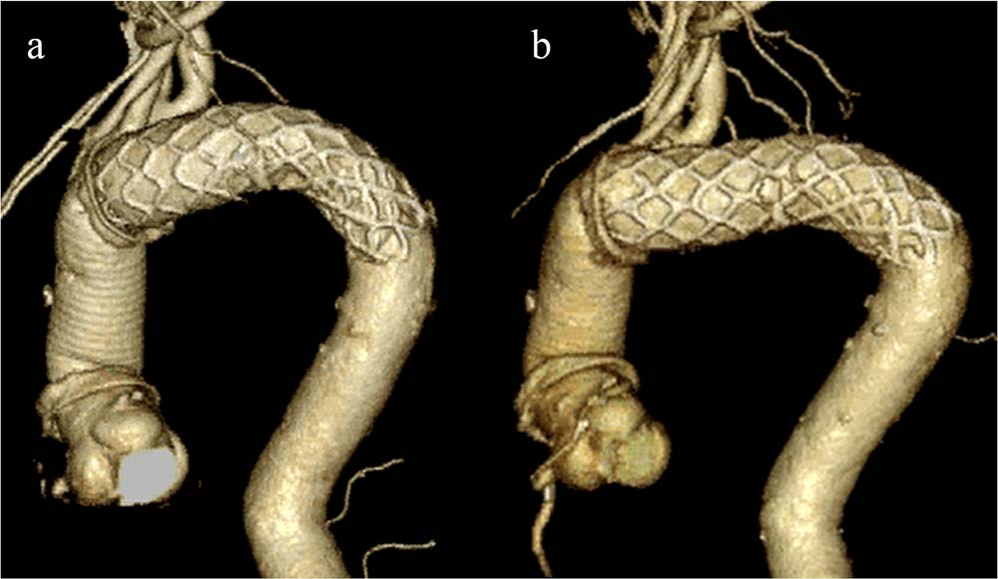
(a) Three-dimensional CT image 1 year after total arch replacement with a FET. (b) Three-dimensional CT image 2 years after total arch replacement with a FET demonstrating straightening and migration of the FET.
